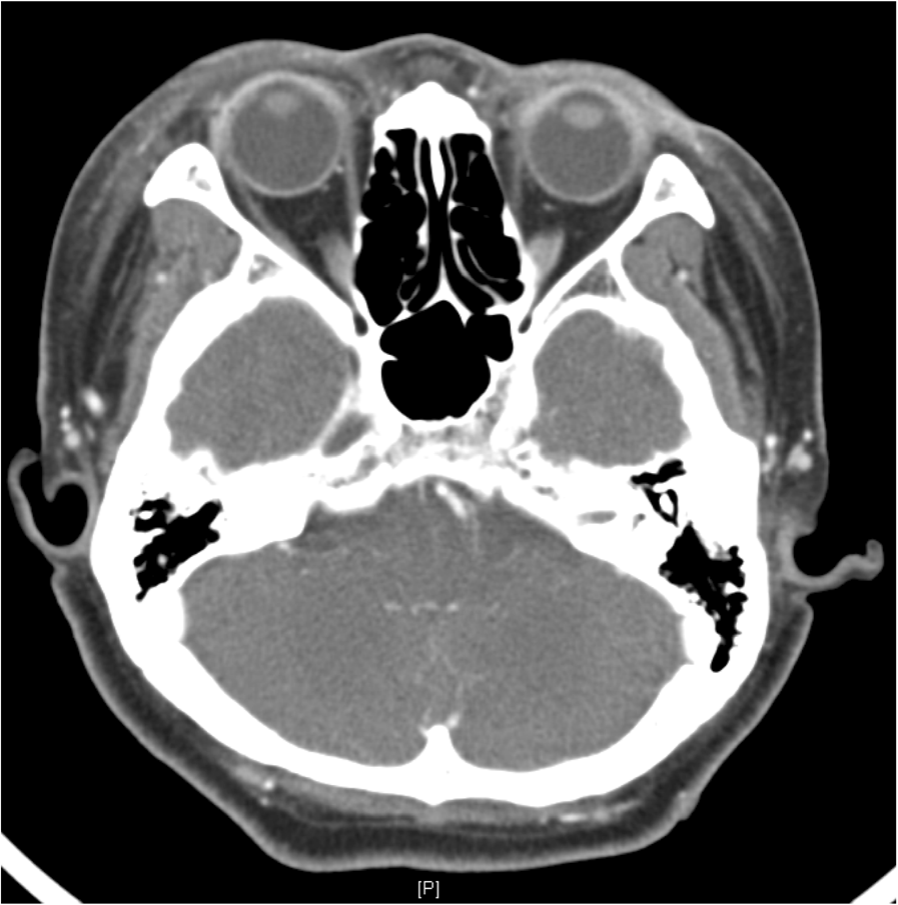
Facial computed tomography image showing no signs of inflammation near the orbits
